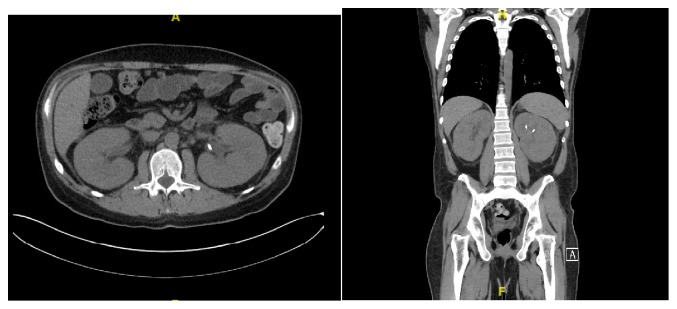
(a), (b) Computed tomography scan showing symmetric enlargement and hypoattenuation of bilateral kidneys with associated fat stranding involving the renal sinuses and perinephric fat. Left sided urinary stent in in place. No hydronephrosis or nephrolithiasis.
